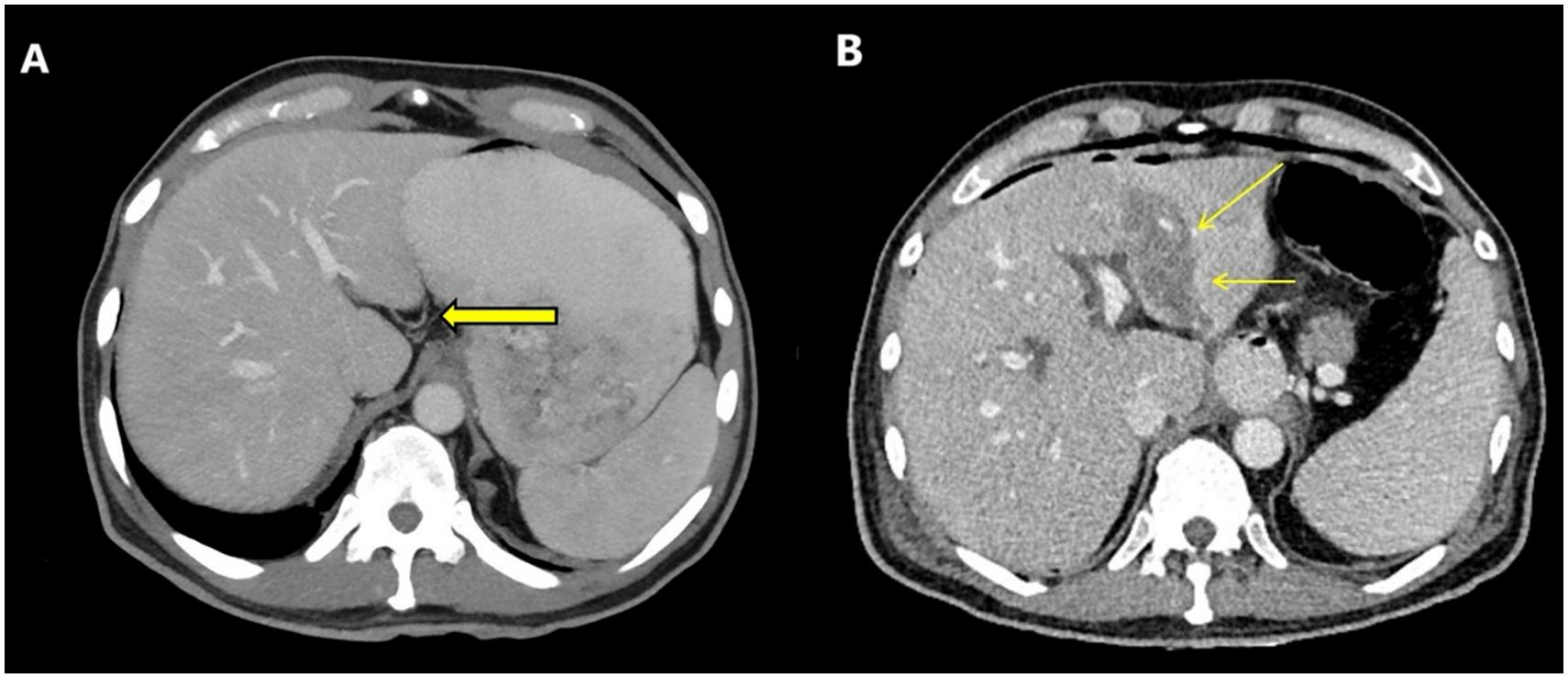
(A) Axial portal phase contrast-enhanced CT MIP image of a preoperative evaluation of a patient with gastric cancer shows the aberrant left gastric vein draining into segment 2 parenchyma (arrow), consistent with Type 1 aberrant left gastric vein. (B) Postoperative abdominal CT of the same patient demonstrates ischemic areas in segments 2, 3, and 4 of the left lobe (arrows).
